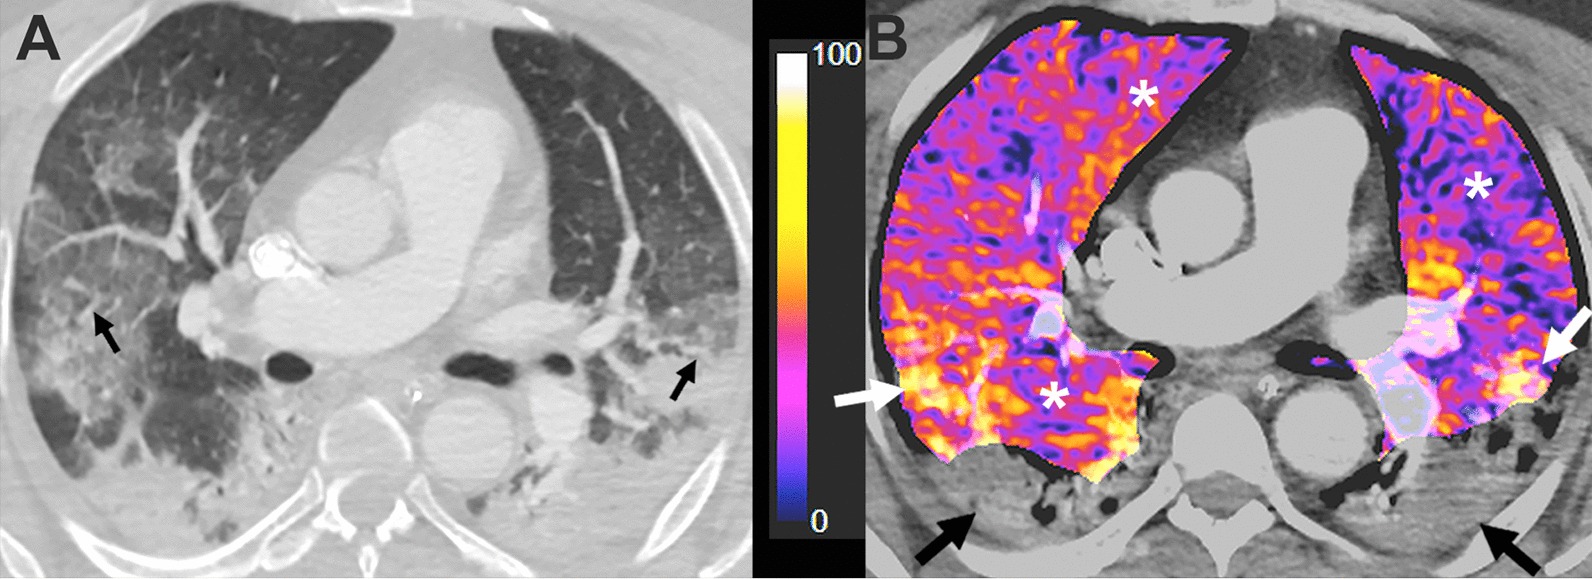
51-year-old male patient, RT-PCR confirmed COVID-19, 3 days since symptom onset. Admission PaO_2_/FiO_2_ ratio was 240, and d-dimer level was 480 ng/mL. Admitted to the intensive care unit, managed with invasive mechanical ventilation. a Axial lung-window CT angiography image shows extensive bilateral ground-glass opacities, with areas of posterior subpleural consolidation. There is subtle subsegmental peripheral vascular dilatation of pulmonary arterial branches (small black arrows). b 5 mm axial reconstruction of a subtraction iodine map shows slight to moderate hypoperfusion predominantly in areas of non-injured lung (*), and more prominent areas of increased perfusion in relation to the zones of ground-glass opacities (white arrows). Perfusion was not assessed in the areas of consolidation (black arrows) due to software limitations that exclude these zones. This patient evolved in a similar fashion to the H (type 2) phenotype described by Gattinoni et al.
